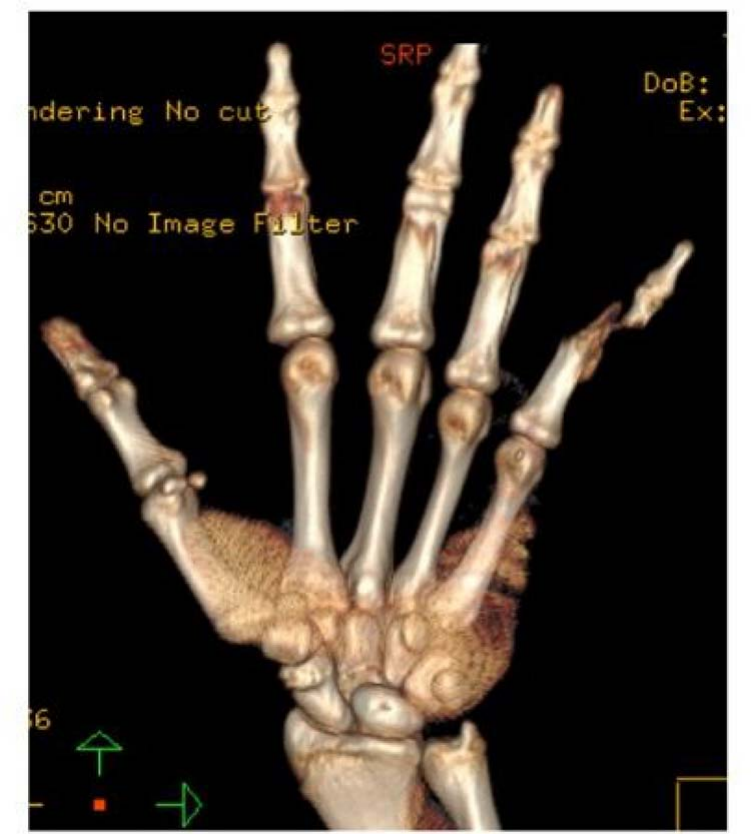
CT scan of the left wrist.
